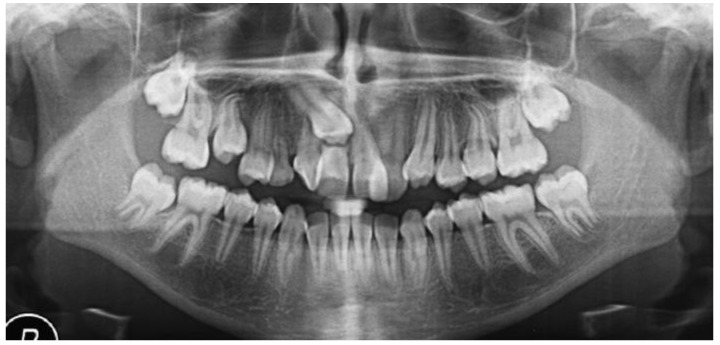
Panoramic radiograph showing transposition of tooth #13 over teeth #11 and #12.
